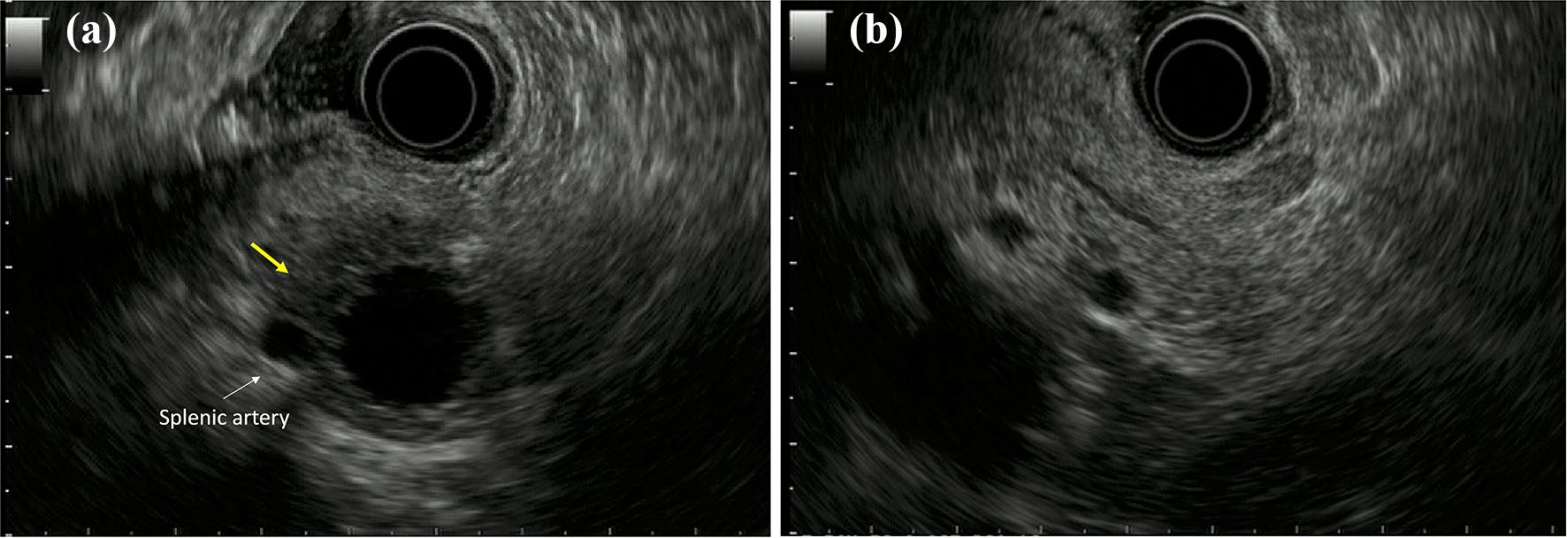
a Endoscopic ultrasound showed partial thickening of the cyst wall and a low-echoic area with indistinct boundaries between the splenic artery and the cyst (yellow arrow). b There were early chronic pancreatitis findings (foci and strand) in the pancreatic parenchyma around the cyst
